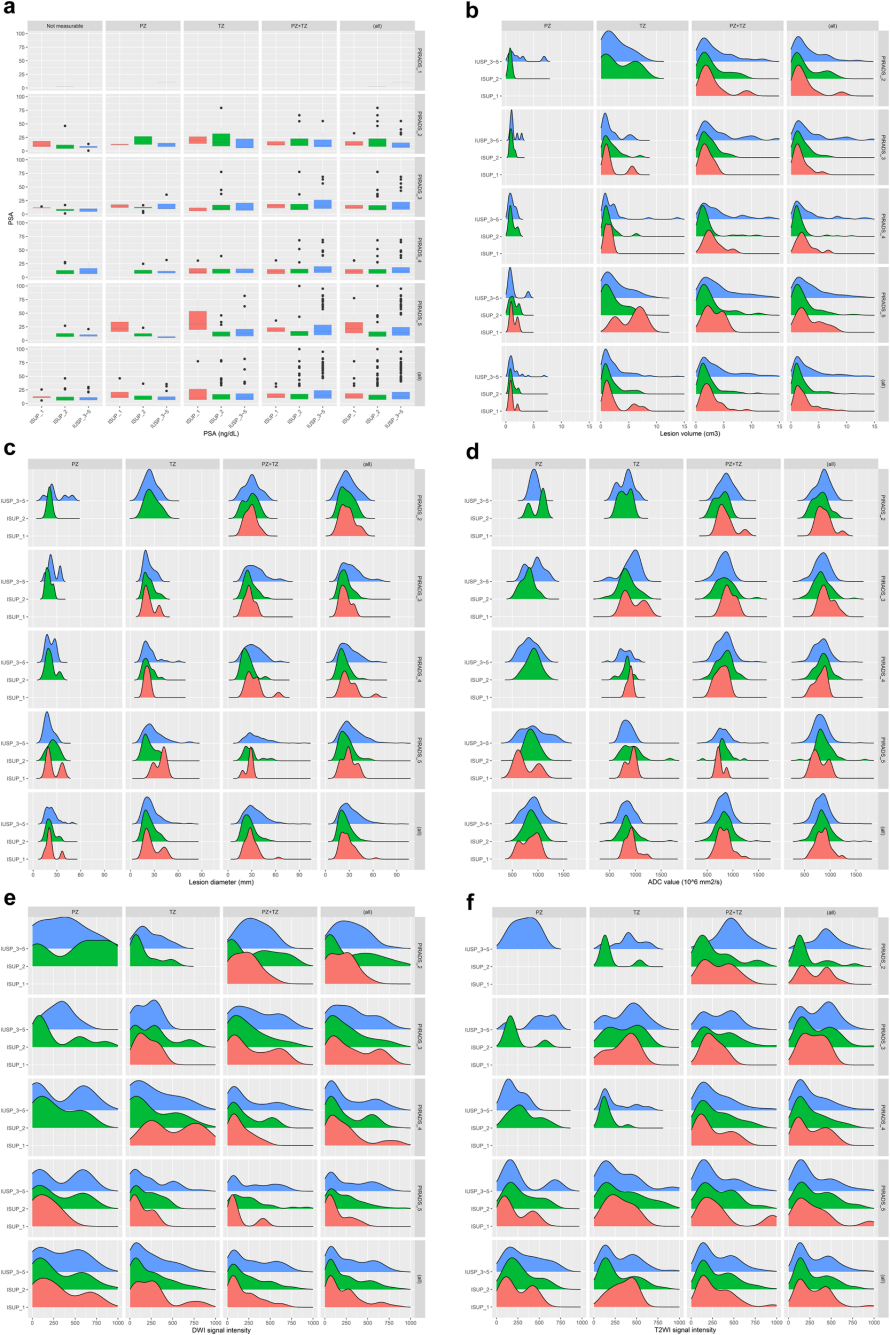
Distribution of the clinical variables among the different ISUP classes. a displays the distribution of PSA levels. Columns 1 to 4 show the PSA values for lesions classified as “not measurable,” lesions in the PZ, lesions in the TZ, and lesions in both the PZ and TZ, respectively. Column 5 displays the PSA values for all lesions. Rows 1 to 5 display the PSA values for lesions categorized as PIRADS_1 to PIRADS_5, and row 6 shows the PSA values for all lesions. b to f demonstrate the distribution of measurements for lesion volume, lesion diameter, ADC value, DWI signal intensity, and T2WI signal intensity, with the similar manner. Columns 1 to 3 display the measurements for lesions in the PZ, lesions in the TZ, and lesions in both the PZ and TZ, respectively. Column 4 represents the measurements for all lesions. When observed by rows, rows 1 to 4 show the measurements for lesions categorized as PIRADS_2 to PIRADS_5, and row 5 displays the measurements for all lesions
